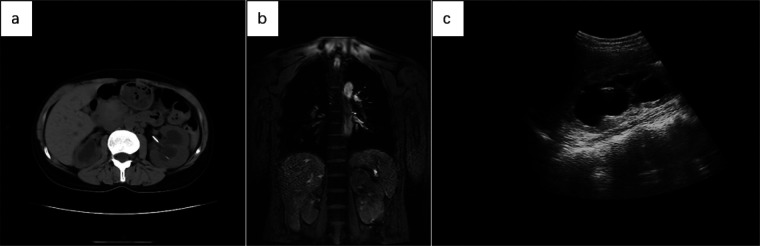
(a) abdominal CT showed increased volume in both kidneys, with more pronounced enlargement of the left kidney and migration of the left renal pelvis following ureteral stent placement. (b) The coronal MRI T2WI of the chest and upper abdomen showed irregular morphology, increased volume, and prominent findings in the left kidney. (c) USG revealed significant expansion of the left renal pelvis, with hypoechoic areas and a thinning renal cortex.
